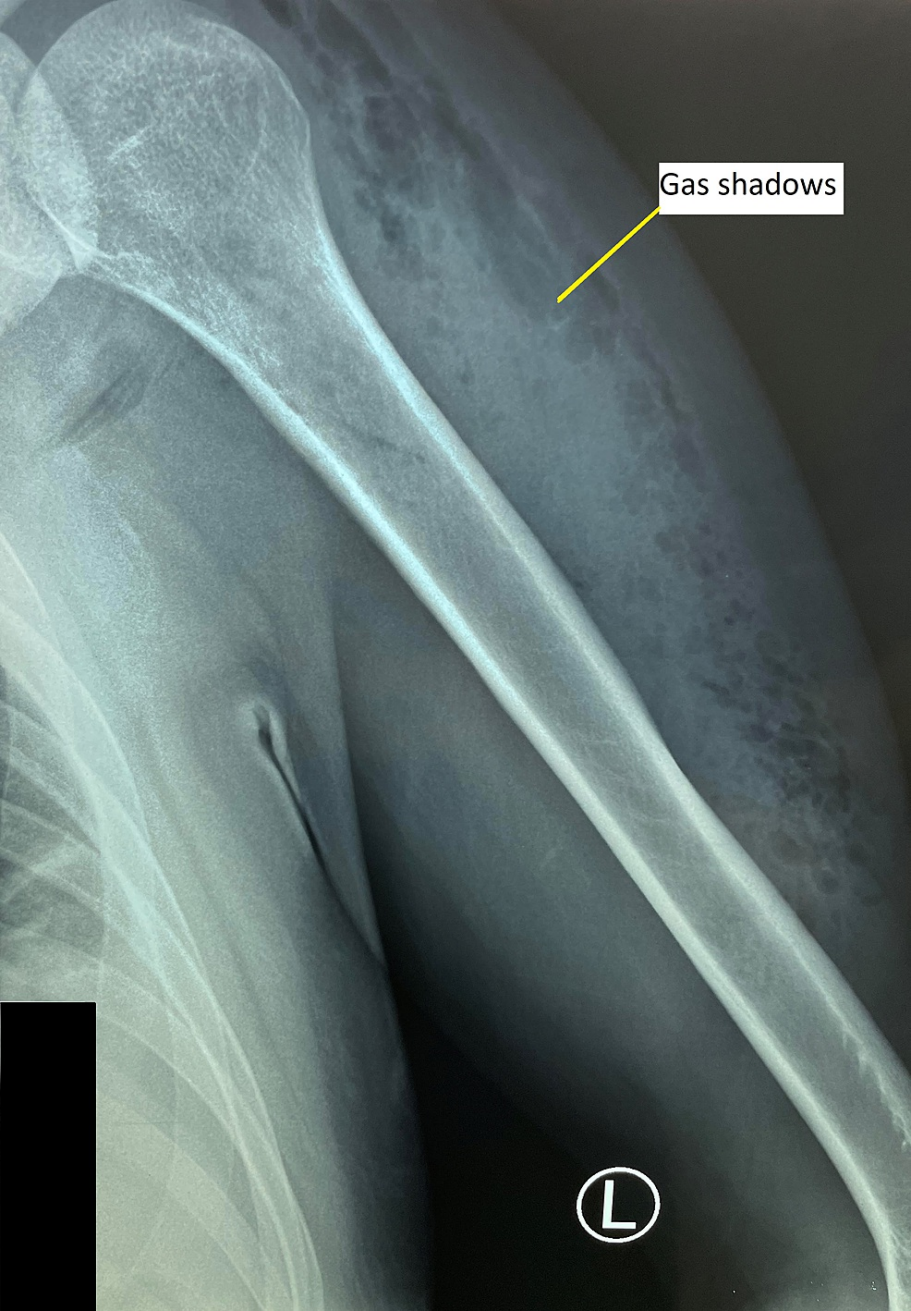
Plain radiography of the left humerus showed presence of gas shadow over the left deltoid region extending along the lateral and posterior aspect of left arm.
